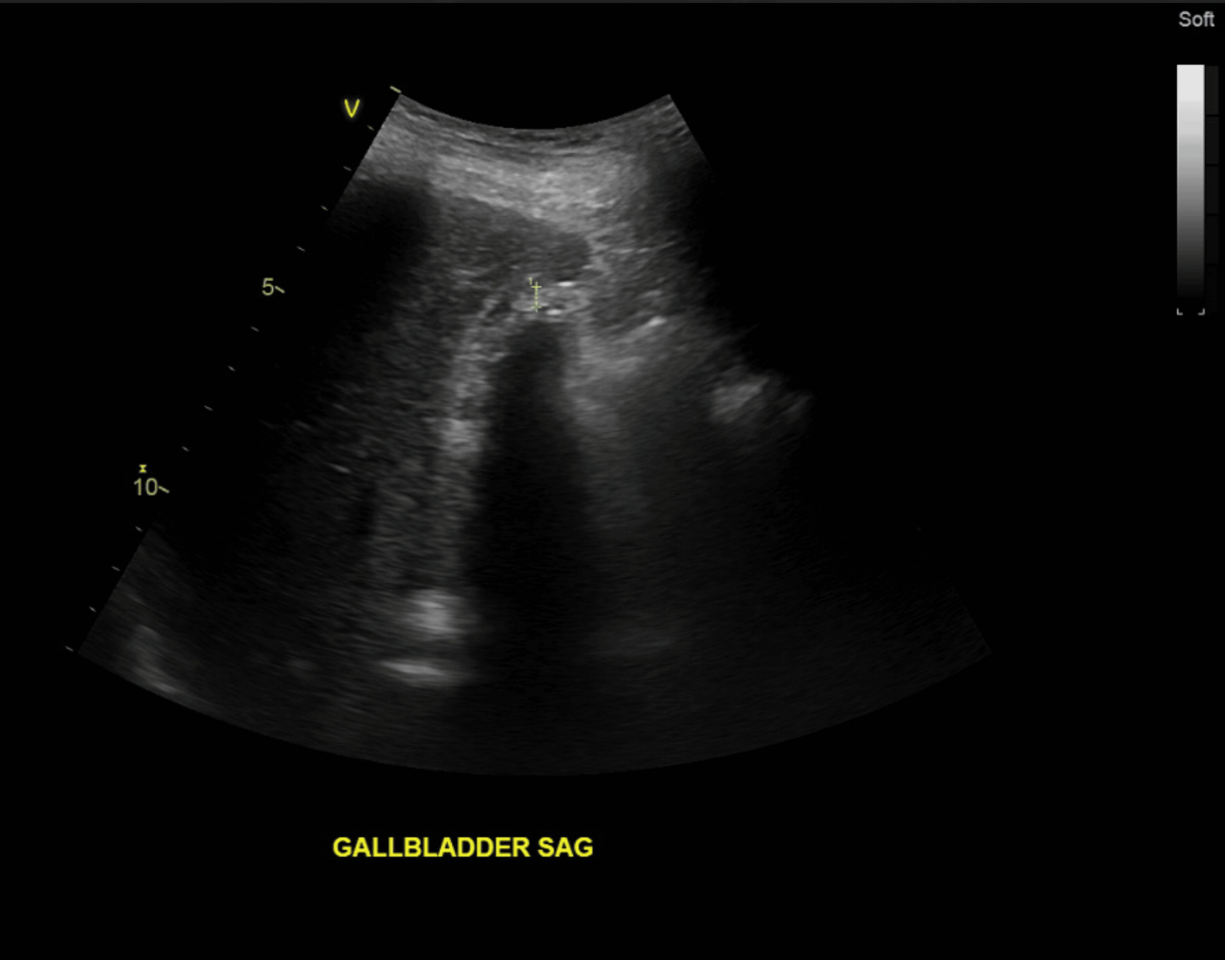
Ultrasound of the gallbladder showing a wall-echo-shadow sign Abdominal ultrasound, sagittal view of the gallbladder, obtained with a curvilinear transducer, demonstrating the wall-echo-shadow (WES) sign consistent with cholelithiasis.
